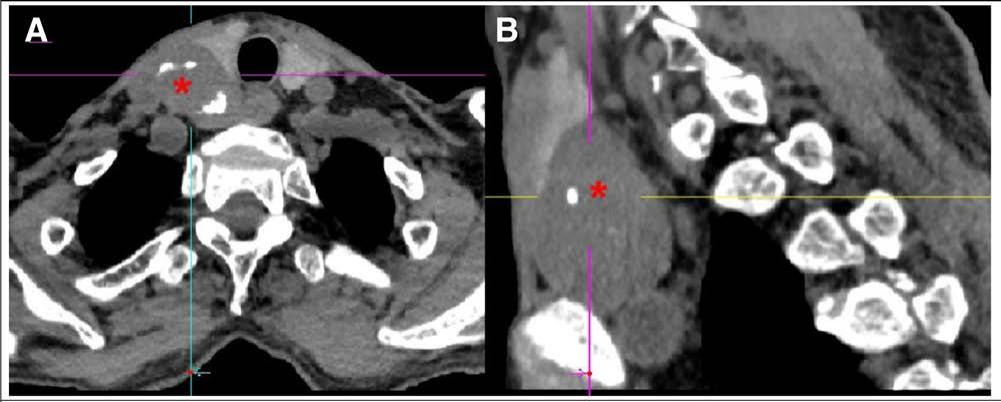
(A) and (B) The neck and pulmonary transversal and sagittal CT scan showed a solid mass below the right lobe of the thyroid (red asterisks). CT = computed tomography.
